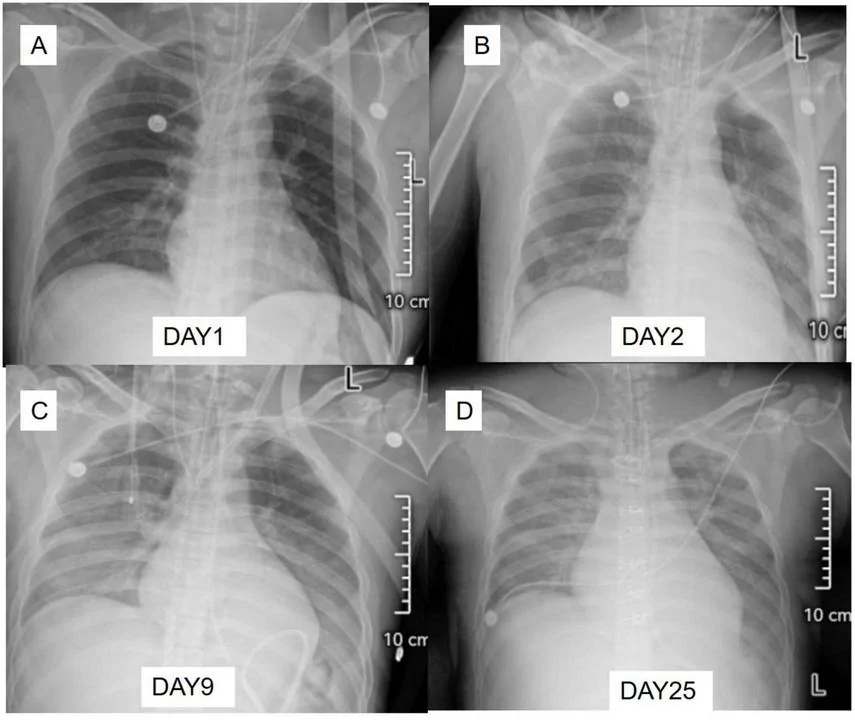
Serial chest radiographs. (A) Pre-VV-ECMO (day 1) showing diffuse bilateral pulmonary infiltrates, indicating severe lung involvement. (B) Day 2 revealing persistent bilateral infiltrates with worsening consolidation. (C) Day 9 demonstrating partial resolution of consolidation and reduction in pulmonary infiltrates. (D) Day 25, post-VV-ECMO weaning, showing significant improvement in lung fields with resolved consolidation.
